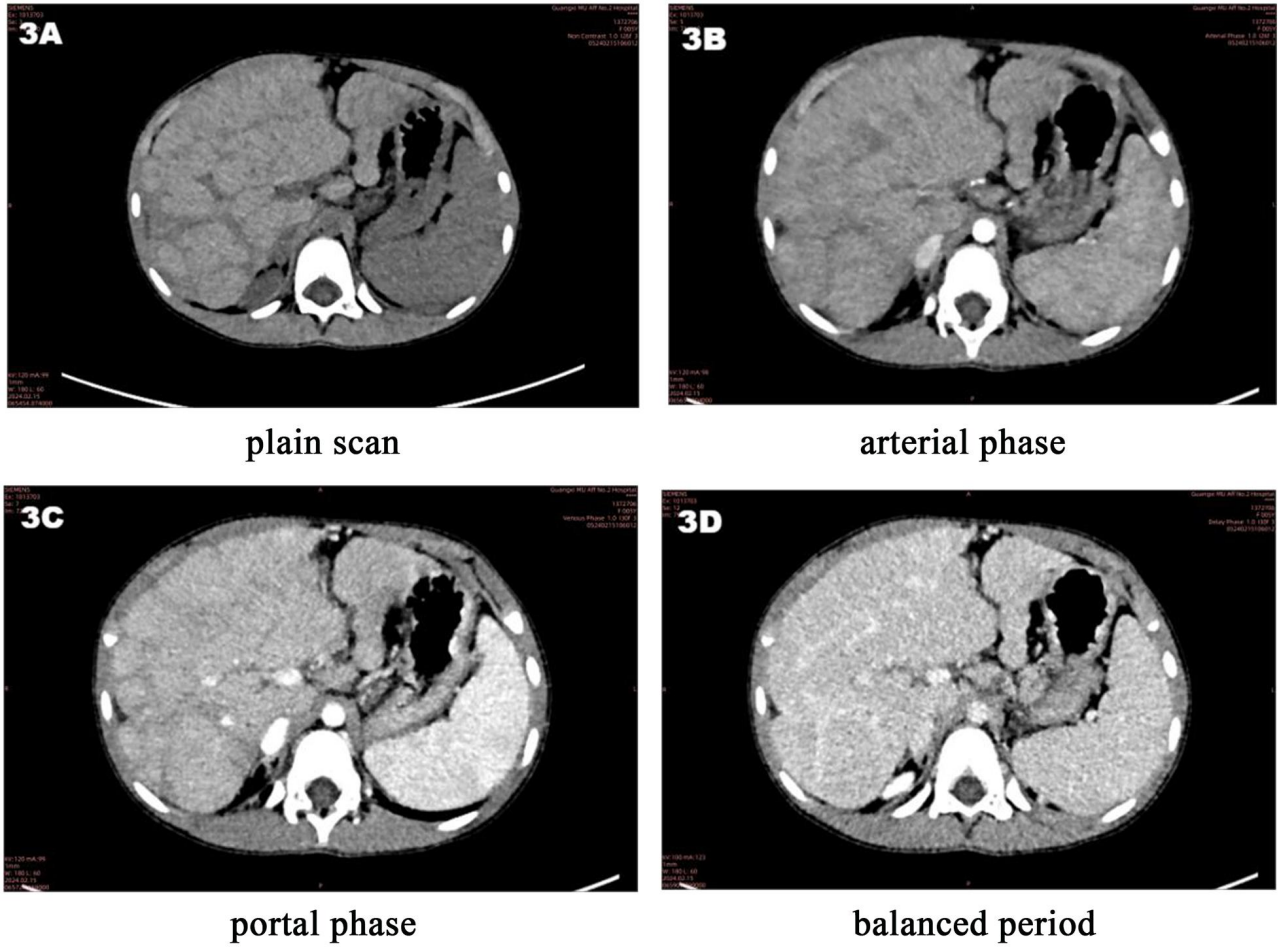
Full abdominal CT plain scan + enhancement: cirrhosis, splenomegaly. (A) Plain scan of the abdomen. The image shows irregular contour of the liver, reduced volume of the right hepatic lobe, and increased density of the liver parenchyma (marked by white arrows), which are typical imaging signs of early cirrhosis. (B) Arterial phase of abdominal enhanced CT. It shows uneven enhancement of the liver parenchyma. (C) Portal phase of abdominal enhanced CT. The portal vein and its branches (e.g., left and right portal veins) are clearly visualized, with no obvious stenosis or thrombosis. (D) Balanced period of abdominal enhanced CT. The liver parenchyma shows relatively uniform enhancement (compared with the arterial phase), and the boundary between the normal and fibrotic areas becomes clearer.
